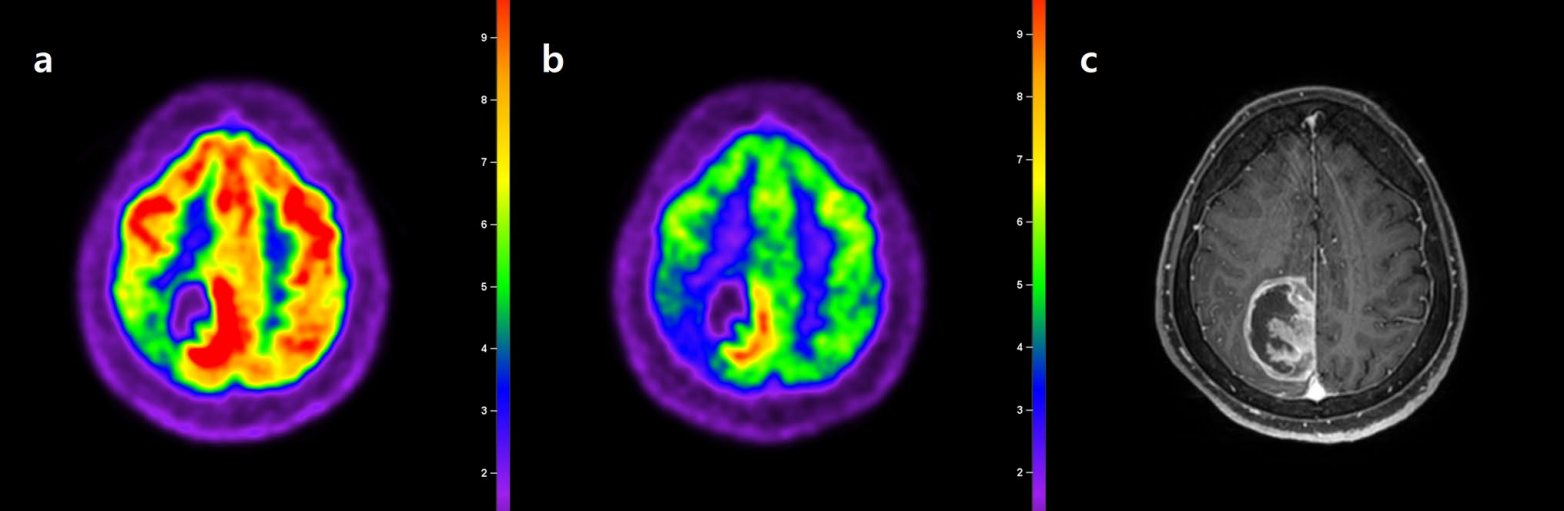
a Transaxial ^18^F-FDG PET, b glucose-loaded ^18^F-FDG PET, and c contrast-enhanced T1-weighted MRI images in a patient with glioblastoma. Due to the high ^18^F-FDG uptake in the normal cerebral cortex, the boundaries of the tumor in the para-sagittal area are not clearly distinguishable in the standard ^18^F-FDG PET scan. However, in the glucose-loaded ^18^F-FDG PET scan, the ^18^F-FDG uptake in the normal cerebral cortex is reduced, allowing for a clearer observation of the tumor’s ^18^F-FDG uptake boundaries
